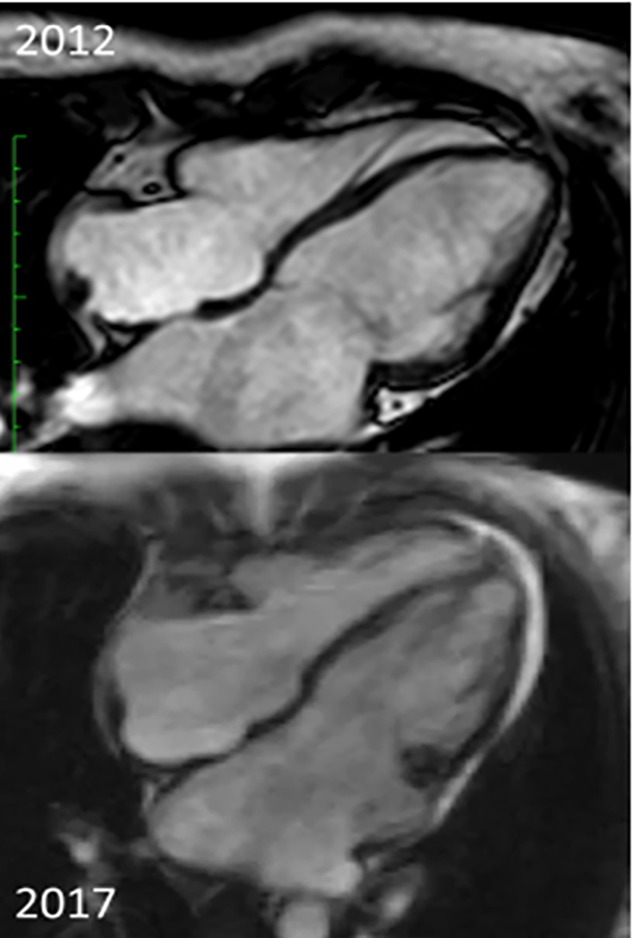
A comparison between cardiac MRI performed in 2012 and 2017
